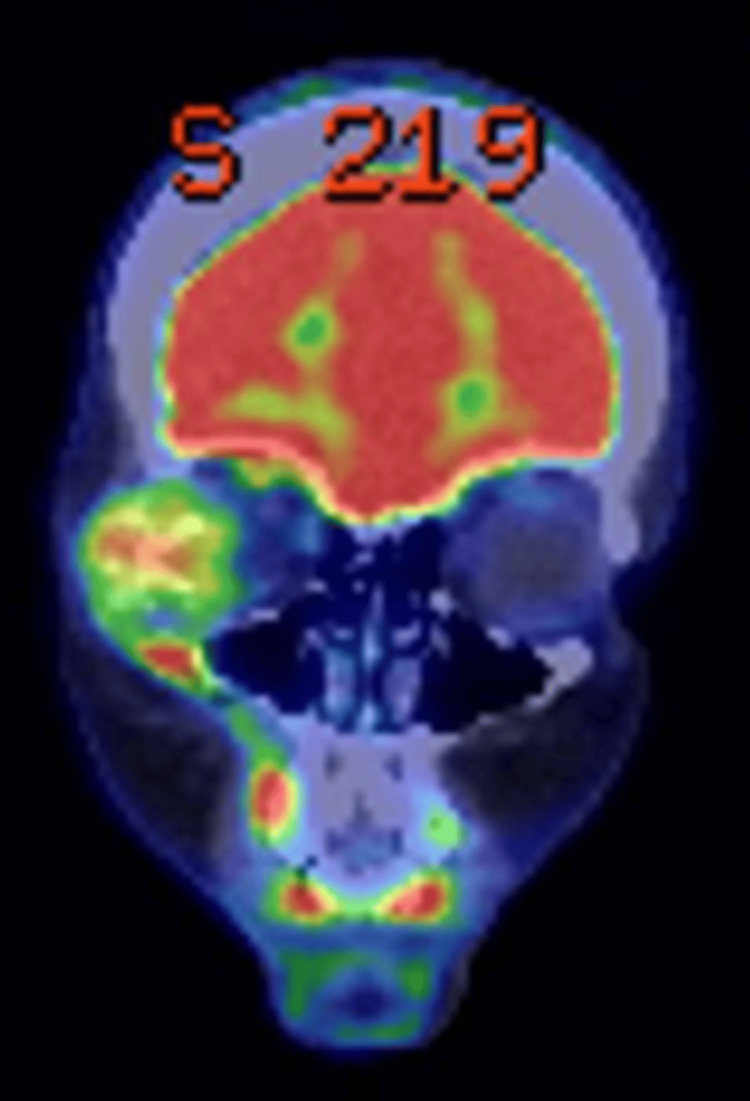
PET-CT demonstrating the metabolically active expansile mass at the right orbit PET: Positron emission tomography
